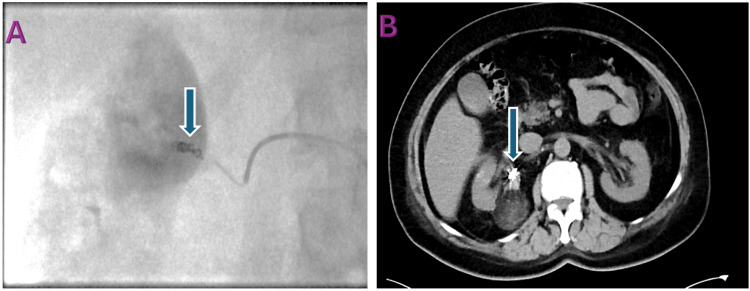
Intraoperative and post-procedure imaging. (A) Intraoperative fluoroscopic angiography: Blue arrow indicates the micro nester coil. (B) Postoperative CT angiogram: Blue arrow highlights the embolization coil.
